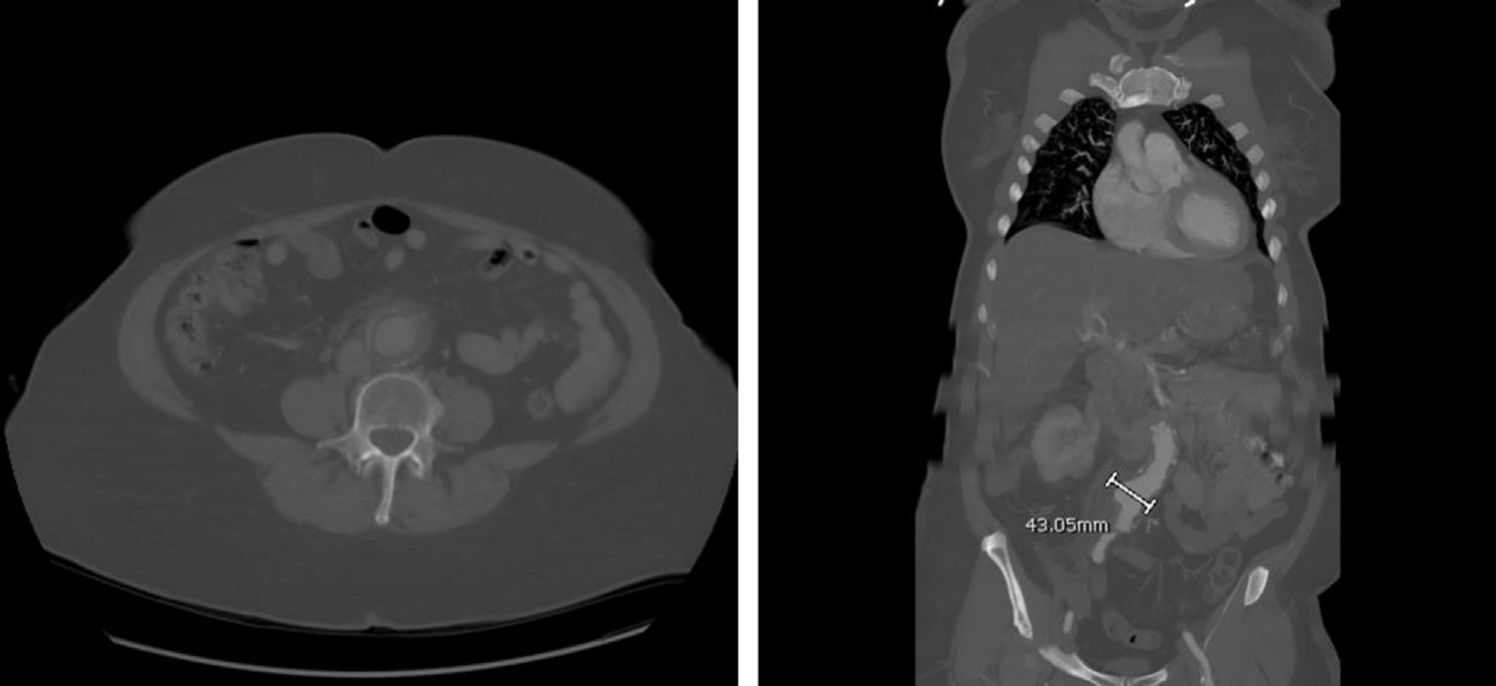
Axial (Left) and coronal (Right) views of initial computed tomography (CT) scan showing a 4.3-cm aneurysmal segment of the abdominal aorta.
